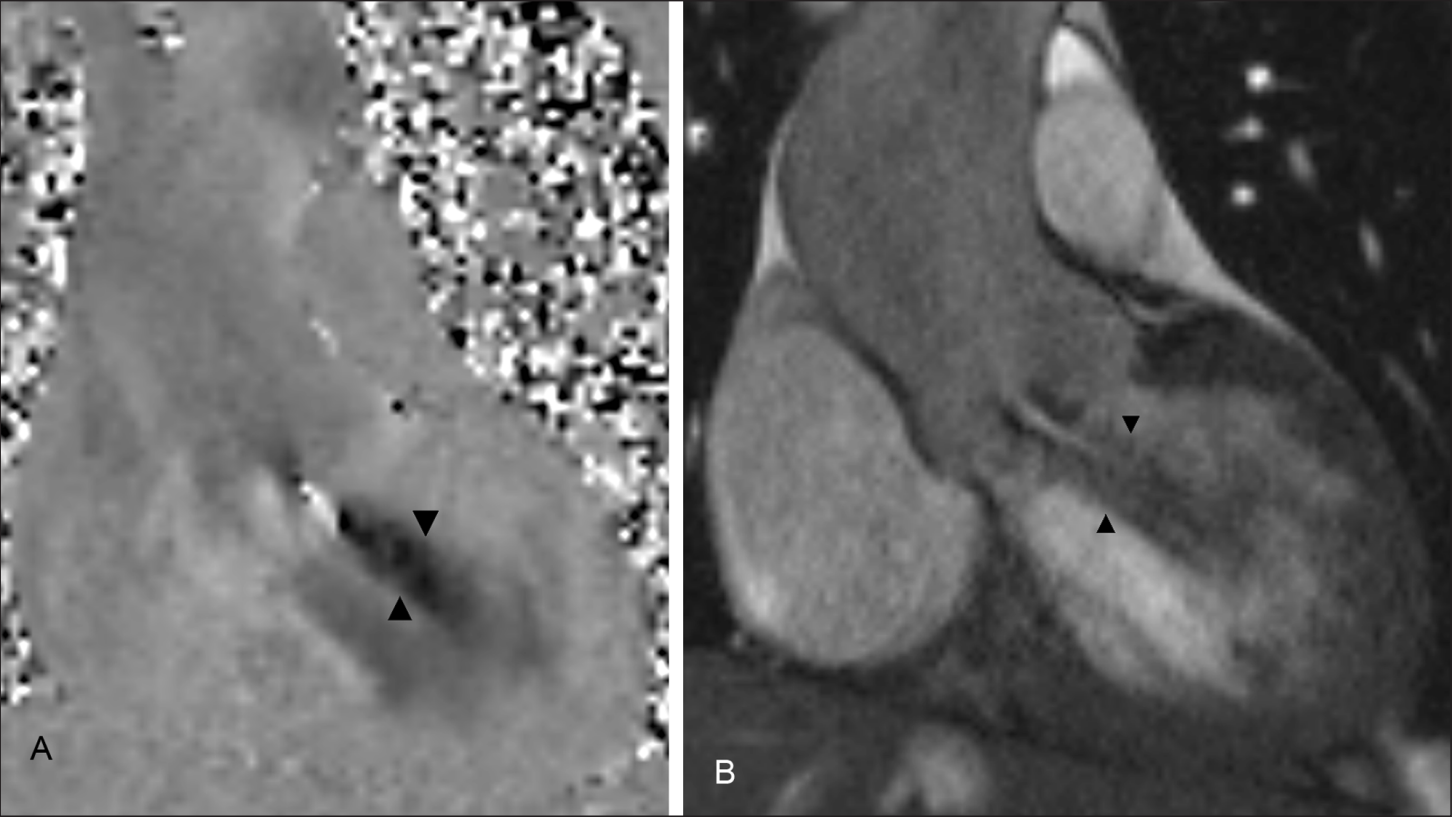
(A) Coronal flow velocity encoded images show holodiastolic regurgitating jet below the aortic valve (arrowheads), with a regurgitation fraction of 49% and regurgitant volume of 81 ml, indicating severe aortic insufficiency. (B) Coronal cine MRI images of the left ventricular outlet during diastole demonstrate the regurgitating jet (arrowheads) during diastole because of aortic insufficiency.
